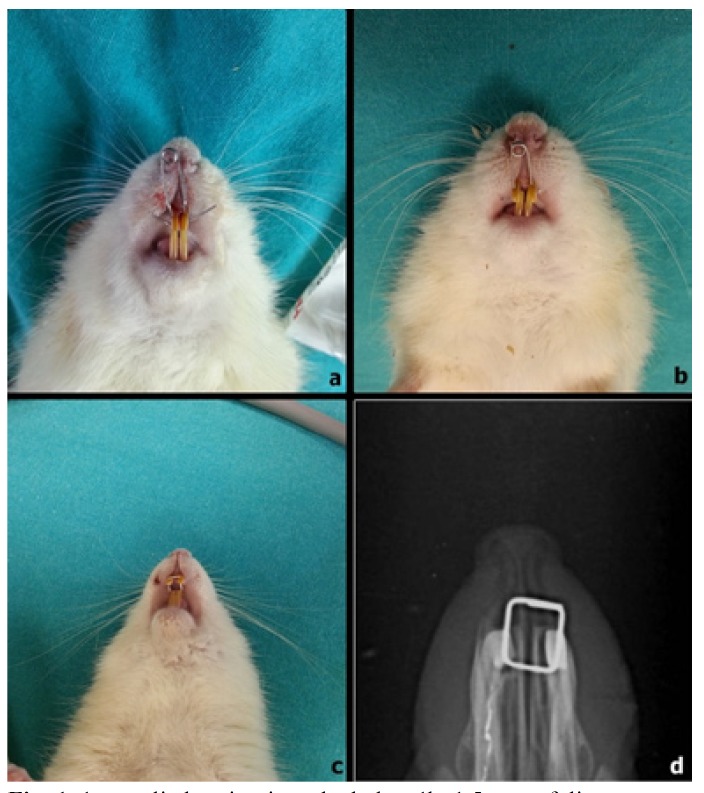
1a, applied spring into the holes; 1b, 1,5 mm of distance was provided after expansion period of 5 days; 1c, applied short lengths of rectangular wires; 1d, occlusal radiographs of the expanded premaxillary suture at the beginning of the retention.
